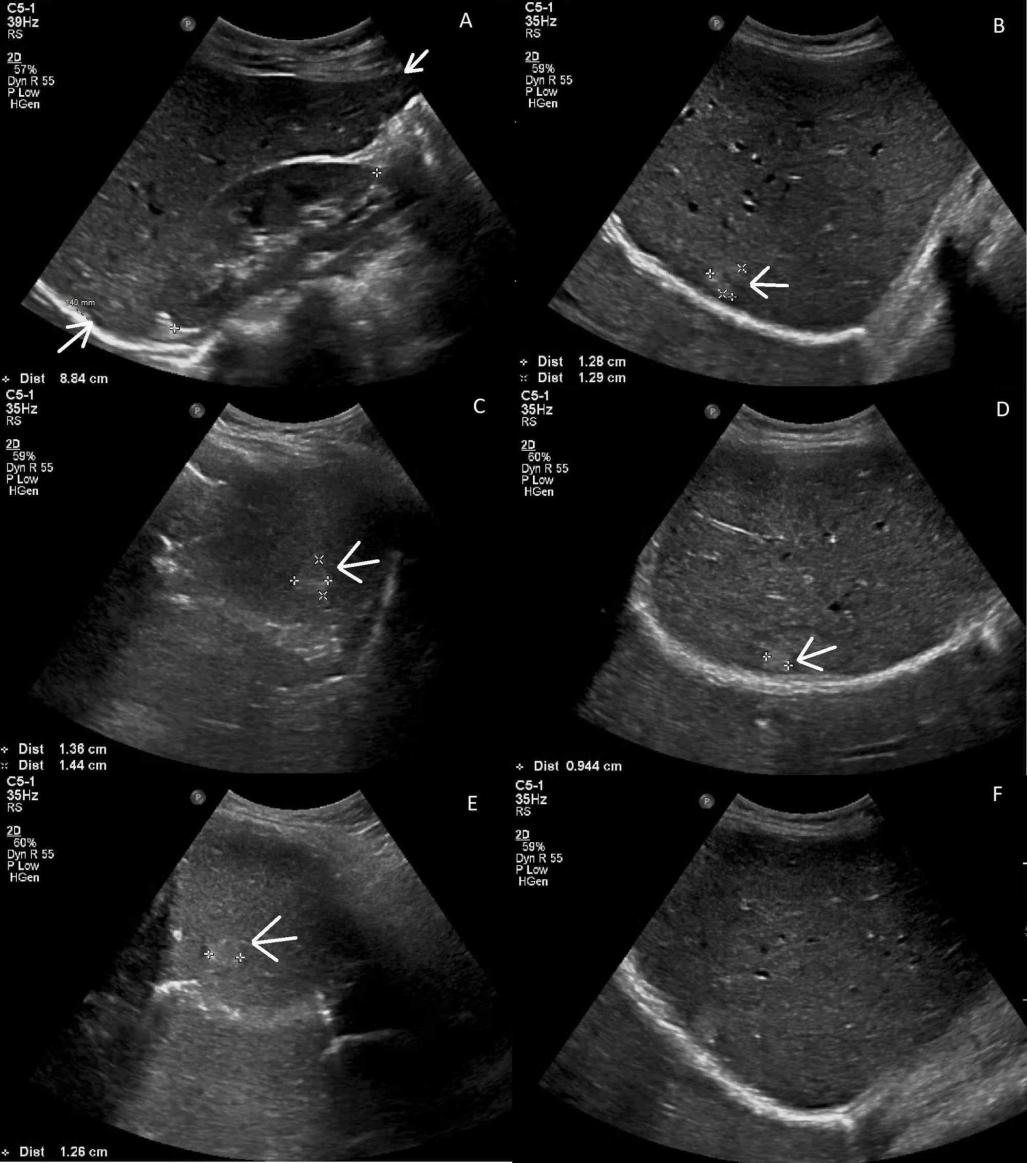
Abdominal ultrasound imaging (A) Two non-specific hepatic lesions. (B, C) 1.4 cm lesion. (D, E) 1.2 cm lesion. (F) Normal liver.
